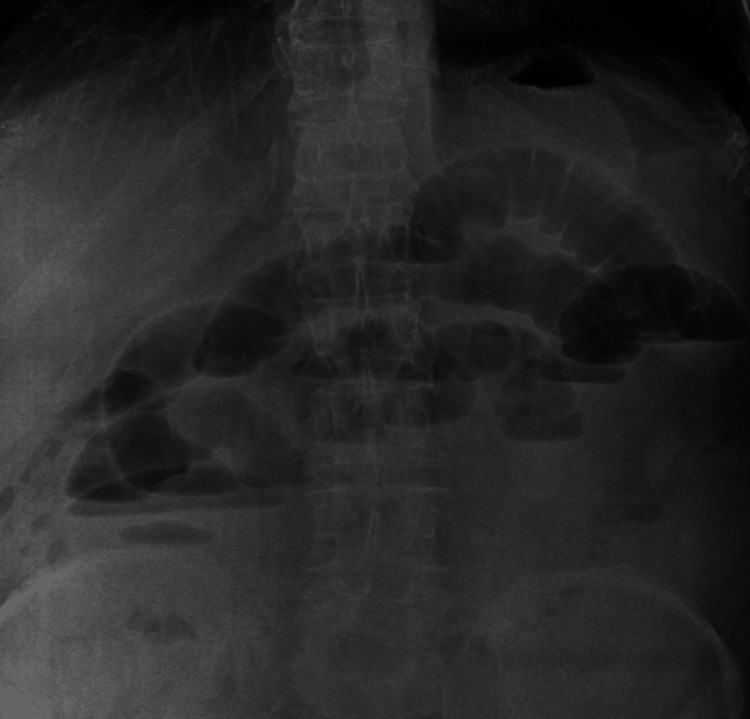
Abdominal X-ray erect image: showing dilated intestinal loops with air fluid levels.
